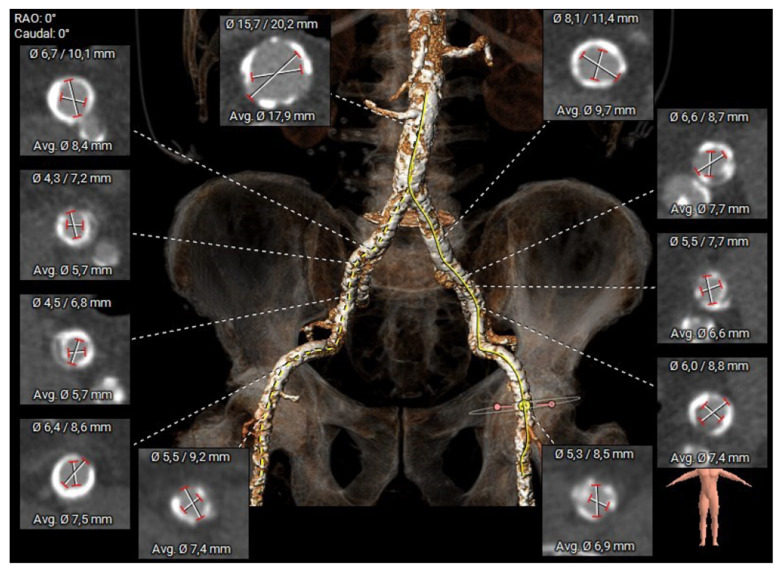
CTA reconstruction of femoral arteries. Calcification load is prohibitive for transfemoral TAVI.
